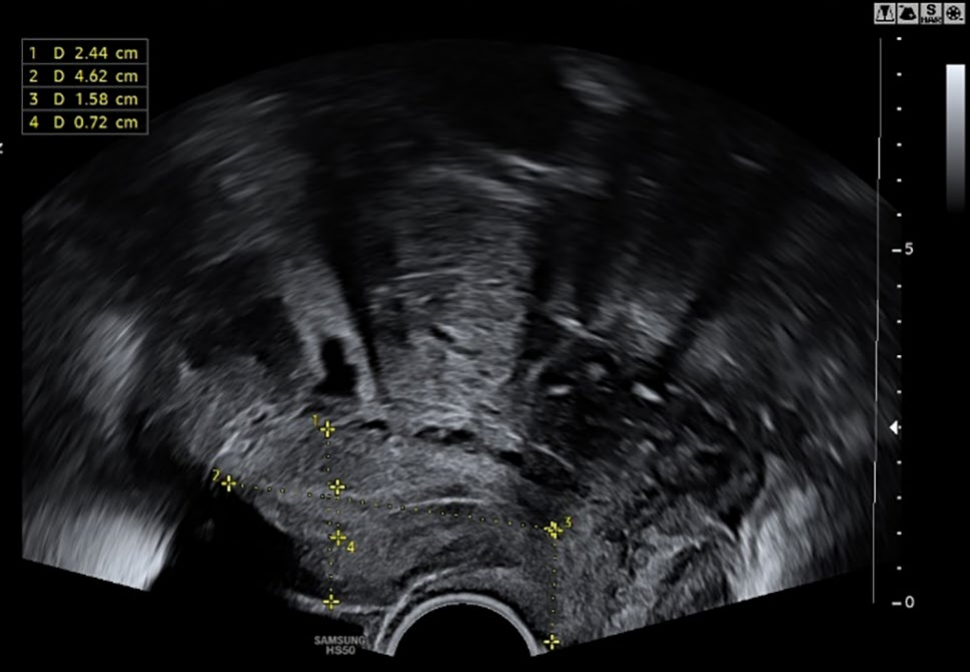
Unremarkable transvaginal sonogram of the uterus
